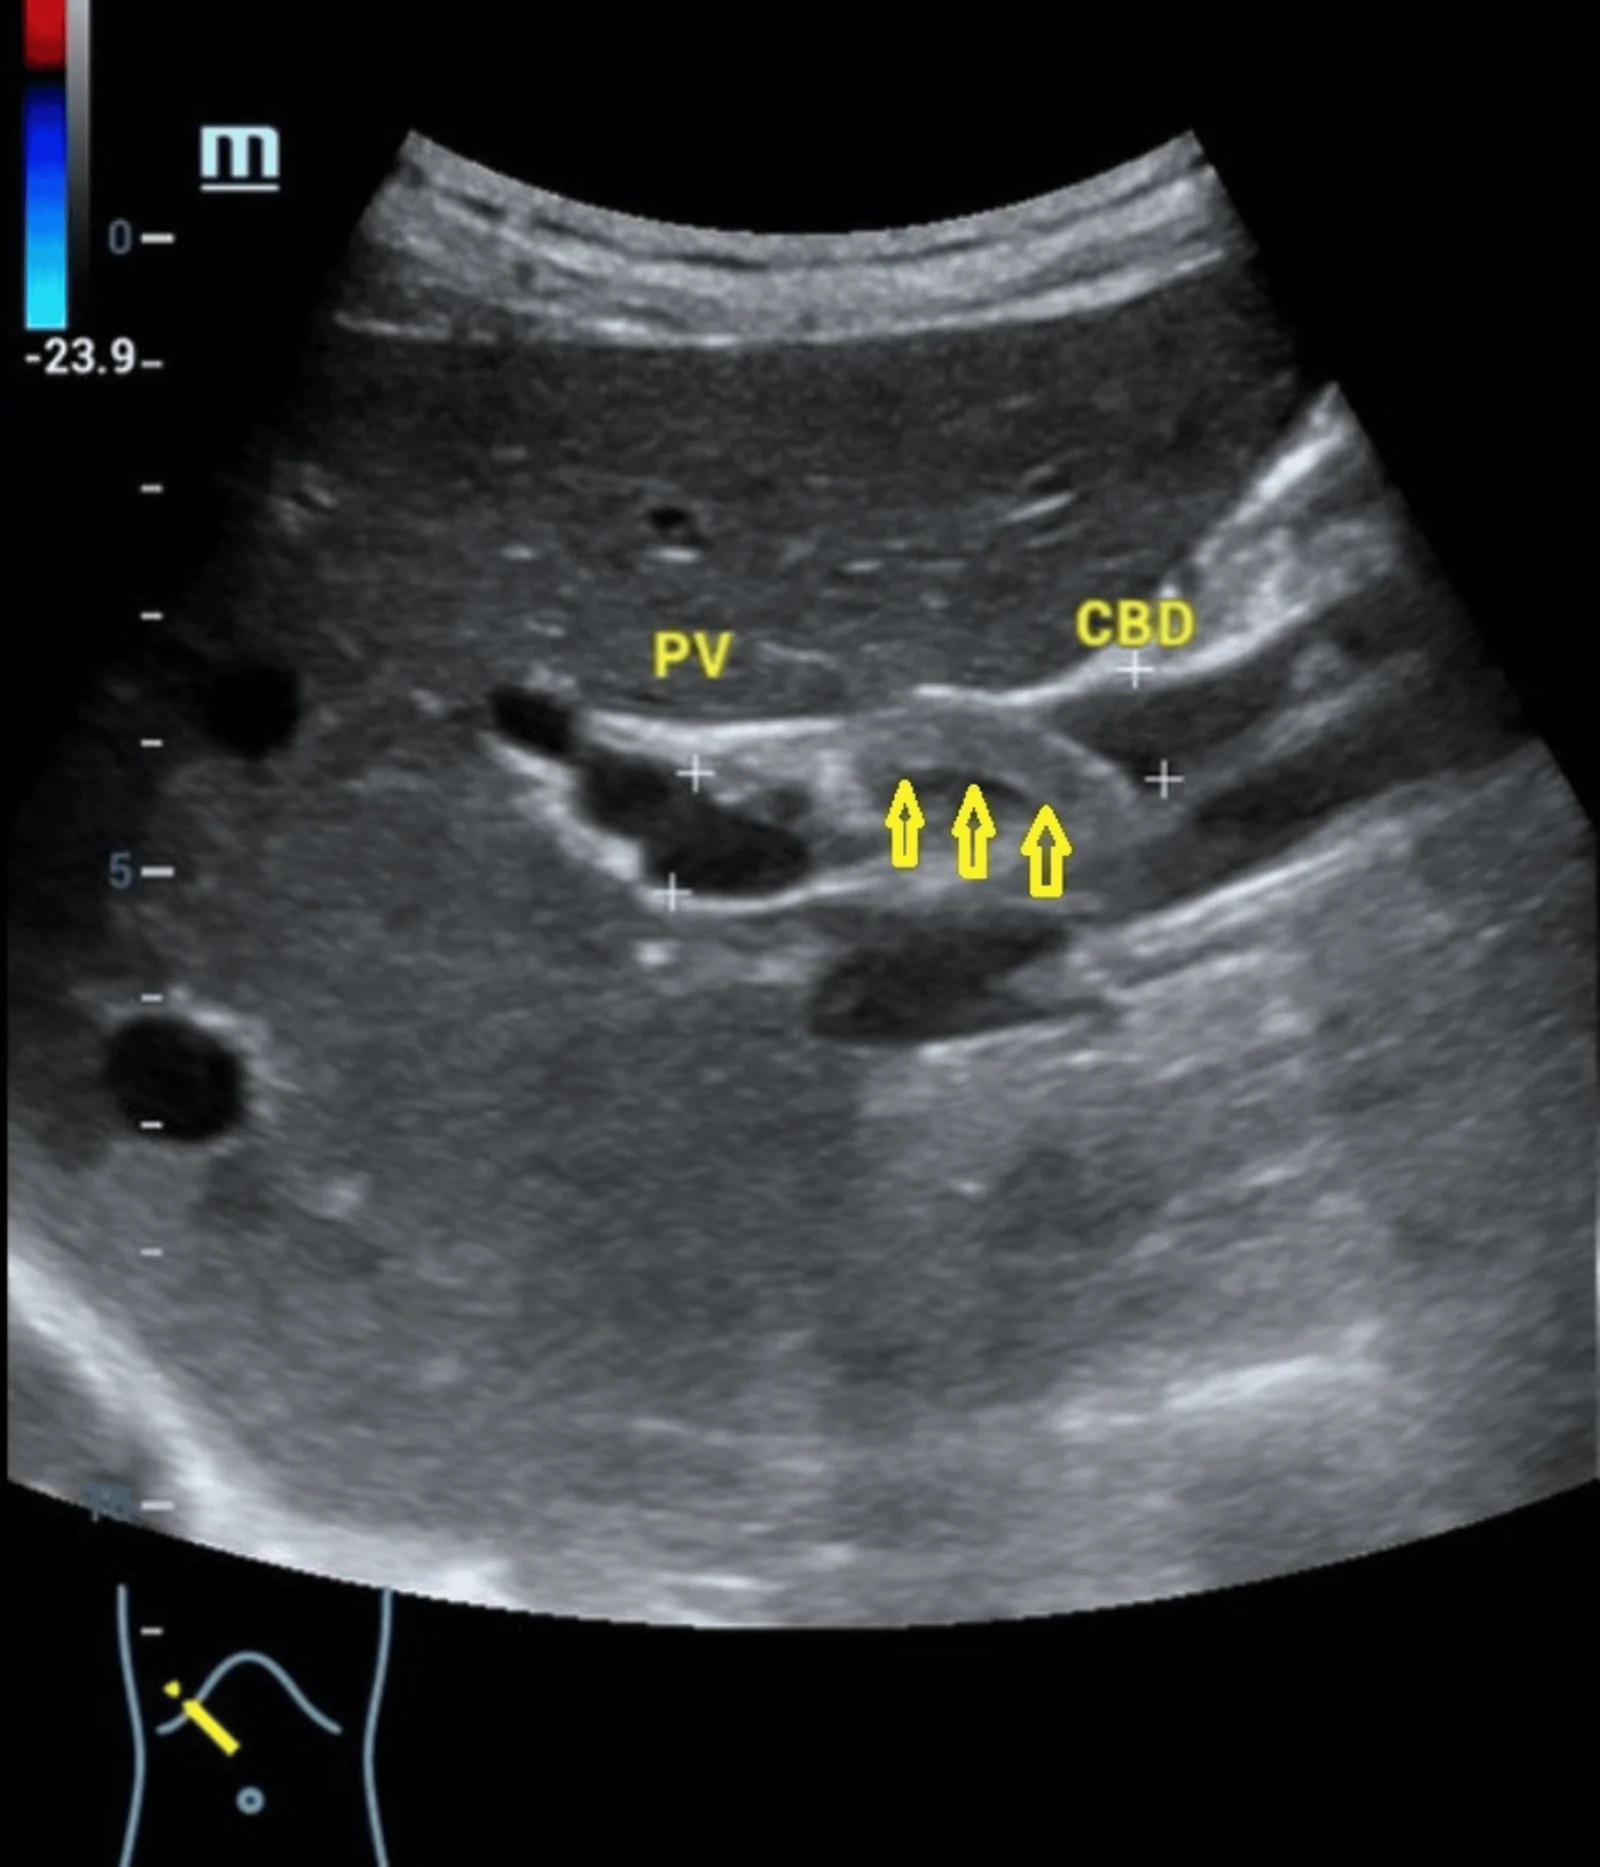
Ultrasound showing tubular hyperechoic structure in CBD The yellow arrows show *Ascaris lumbricodes.* CBD: common bile duct; PV: portal vein
